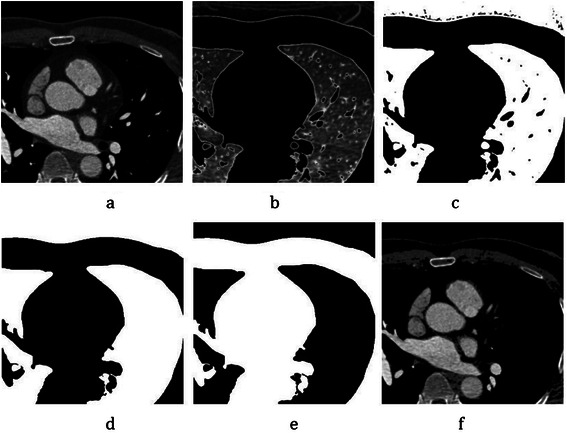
Mask construction for CTA images. (a) Select CTA slice. (b) Extract region 1 based on Hounsfield Unit (*HU*) measurement. (c) Create the binary image by considering specific threshold. (d) Fill holes (for pulmonary vessels). (e) Construct mask. (f) Apply mask on regions 3 and 4 (*HU* ≥ 31).
